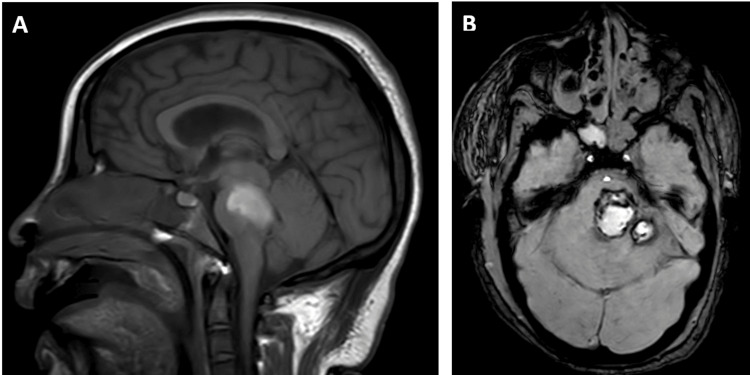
Saggital and axial MRI images. (A) Sagittal T1-weighted MRI showing an extensive hyperintense signal along the midbrain and pons (B) Axial T2*/SWI sequence demonstrating hyperintensity involving the pons, cerebral peduncle, and left cerebellar hemisphere, surrounded by a thin rim of signal void.
